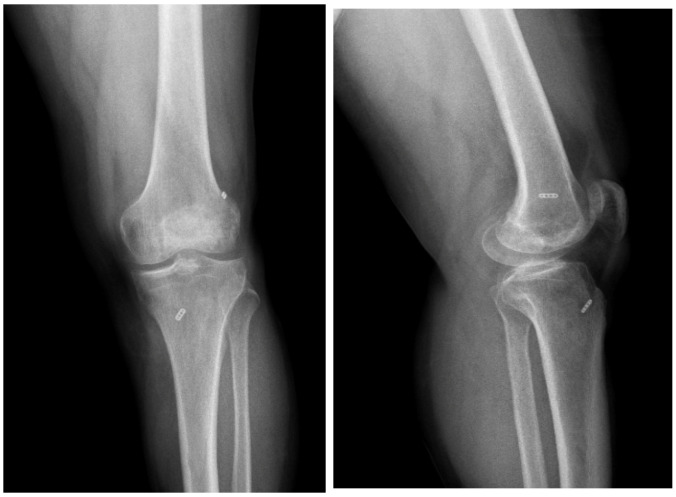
Postoperative AP knee radiographs after all-inside ACL reconstruction.
